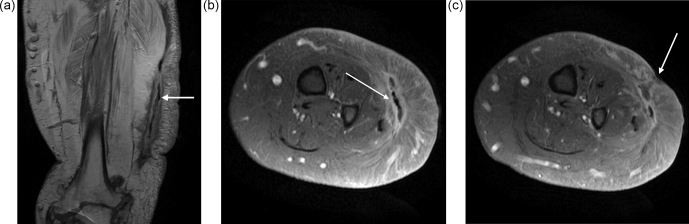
MRI revealed the extent of the infection around the heterotopic bone
